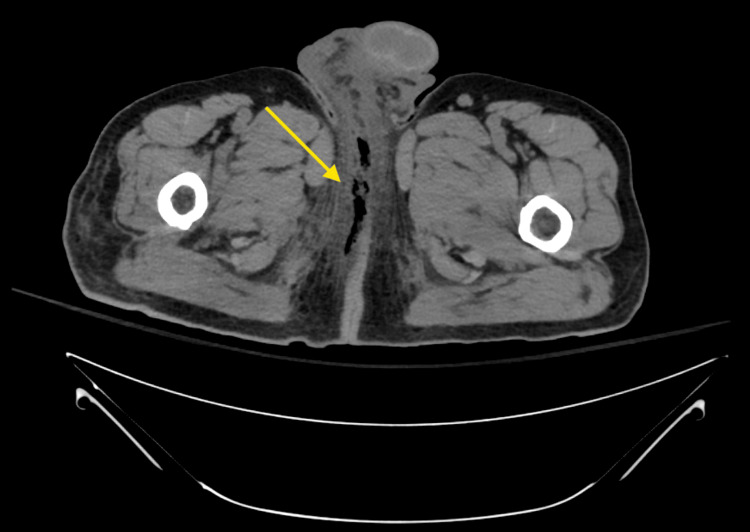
Computed tomography of the abdomen and pelvis
